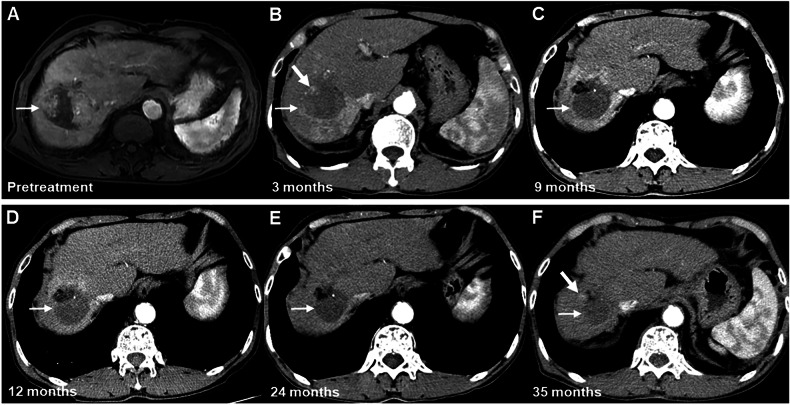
Images of a 76-year-old man with HCC treated by SIRT. MRI or CT obtained during the arterial phase. A Pretreatment contrast-enhanced MRI scan shows a 6.2 cm clinically diagnosed HCC (arrow) at segments 7 and 8 of the liver. B On the posttreatment contrast-enhanced CT scan performed at 3 months after SIRT, masslike enhancement (thick arrow) was seen and decreased in size in the treated lesion (thin arrow), and this lesion was assigned to the LR-TR nonprogressing category. C–E On 9–24-month follow-up CT scans, no mass-like enhancement was seen in the treated lesion (arrows) or along its margin; therefore, this lesion was assigned to the LR-TR nonviable category during this period. F On a 35-month follow-up CT scan, however, there was a 1.1 cm newly appeared masslike enhancement (thick arrow) along the margin of the treated lesion (thin arrow); thus, this lesion evolved into the LR-TR viable category. CT, computed tomography; HCC, hepatocellular carcinoma; LR, liver imaging reporting and data system; MRI, magnetic resonance imaging; SIRT, selective internal radiation therapy; TR, treatment response
